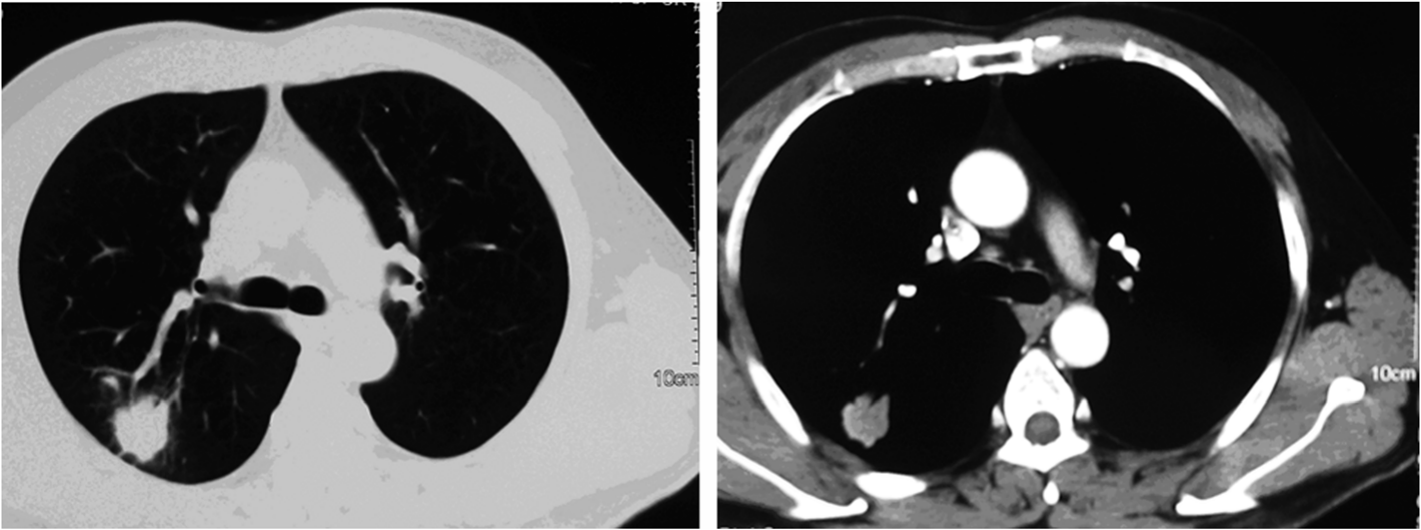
Chest computed tomography scan showing a mass with signs of lobulation and burr, sized 2.4×2.0cm, in the dorsal section of the right lung.
